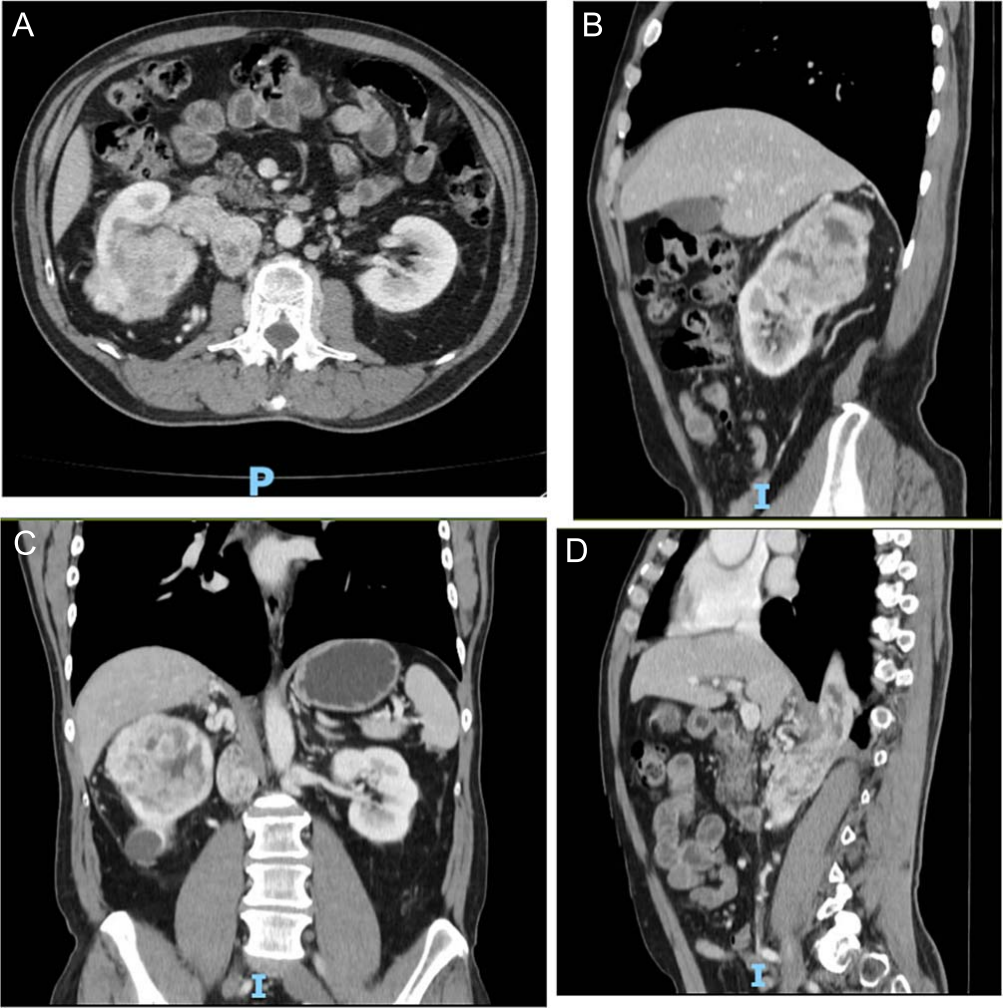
(A–D) Abdominopelvic contrast-enhanced computed tomography prior to neoadjuvant therapy showing a lesion in the right kidney with associated mural thrombus extends through the azygos vein in the sagittal section.
